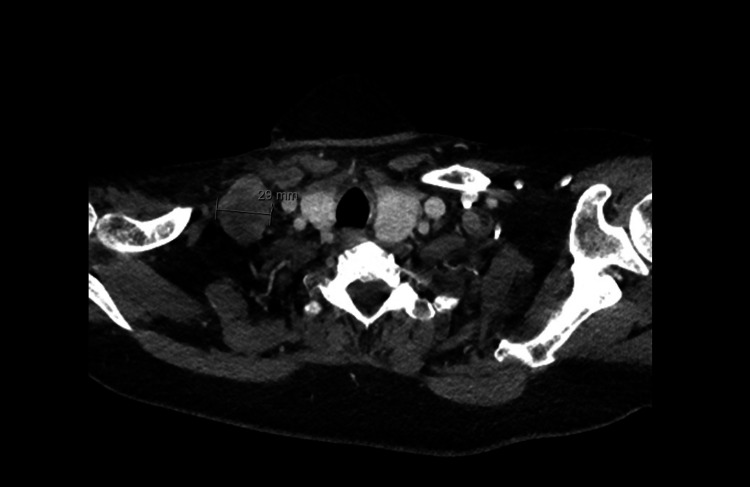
CT neck demonstrating large lymph nodal mass in the right lower cervical region measuring 2.9 cm
